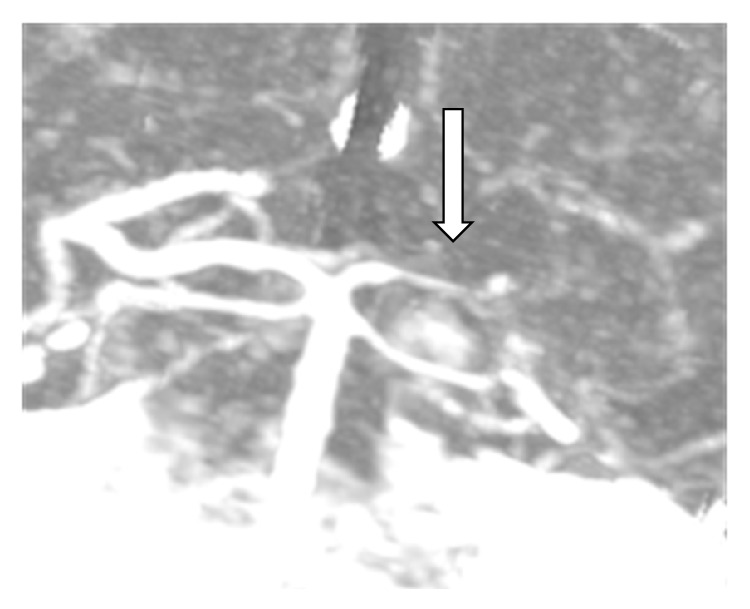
CT angiography shows gracile flow of the right posterior cerebral artery with narrowing of the P1 segment (*white arrow*).
